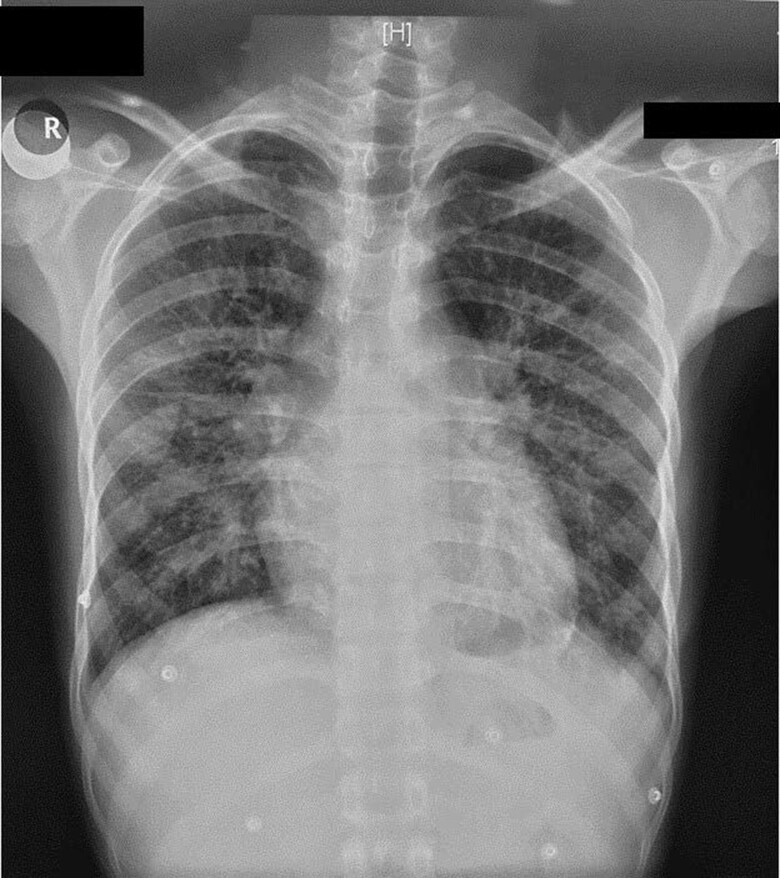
Chest radiograph showing pulmonary venous hypertension.
